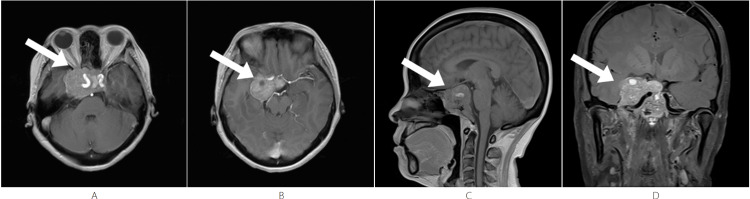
Nine Months Post-IMPT - July 2024 The volume of the sellar mass is slightly reduced compared to the previous scan; the lesion anterior to the left midbrain is smaller; compression of the brainstem and optic chiasm is slightly relieved. (A, B) Axial T1-weighted post-contrast images show that the sellar mass is slightly smaller compared to previous scans; the lesion anterior to the left midbrain is smaller (arrow). (C) Sagittal T1-weighted post-contrast image shows the lesion with heterogeneous mild-to-moderate enhancement (arrow). (D) Coronal T1-weighted post-contrast image shows the lesion with heterogeneous mild-to-moderate enhancement (arrow). IMPT, intensity-modulated proton therapy
